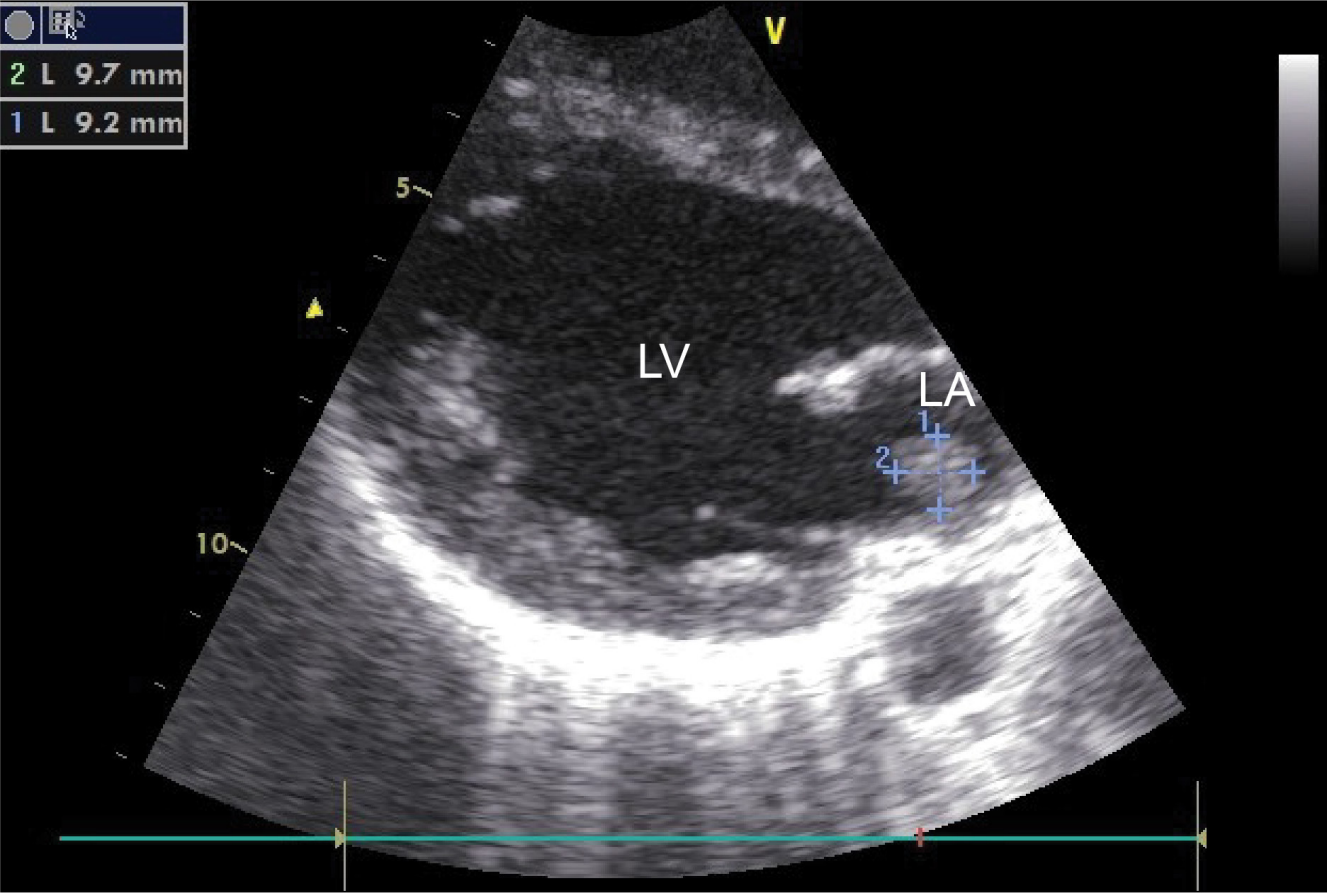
Transthoracic echocardiogram: The verruca was approximately 10 mm in diameter, attached near the posterior mitral valve leaflet and mobile. LA, left atrium, LV, left ventricular.
